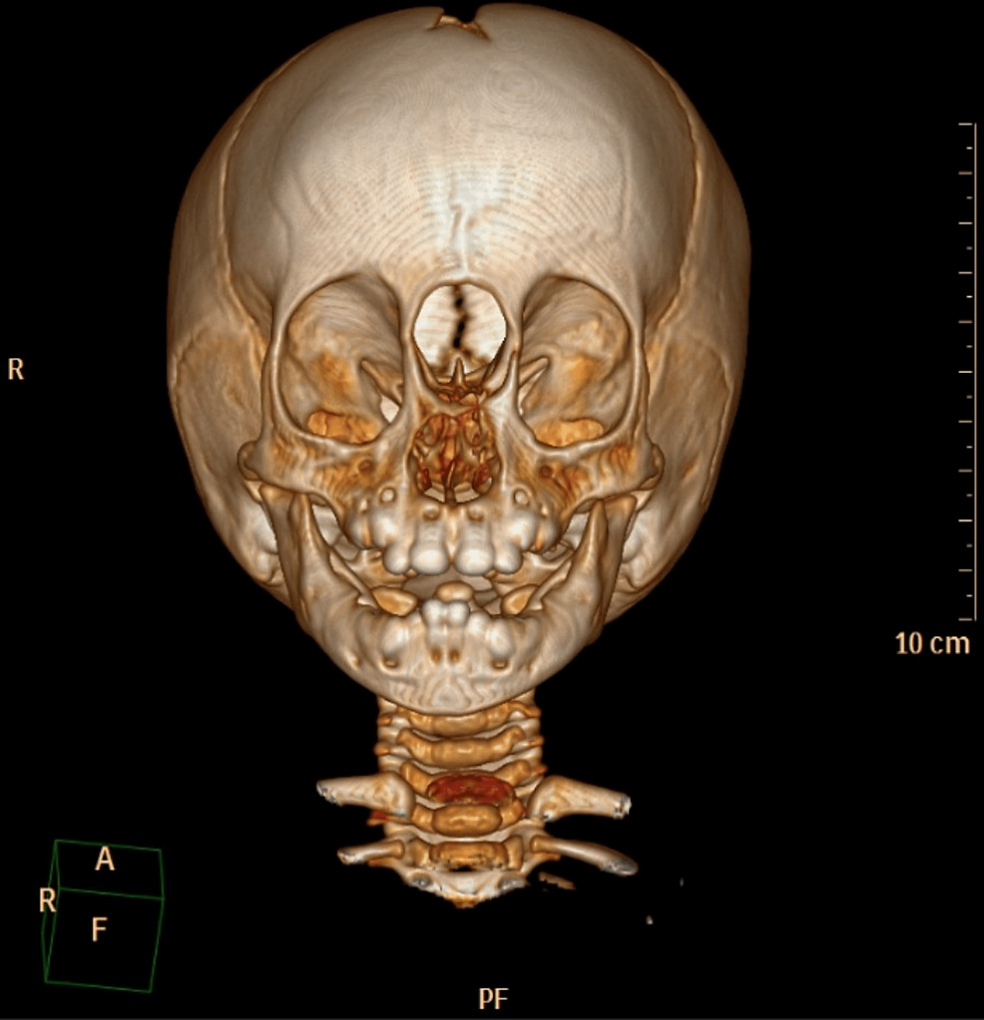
Three dimensional CT reconstruction showing extensive bone defect located at the anterior portion of the skull through which there was herniation of the meninges and brain parenchyma.
